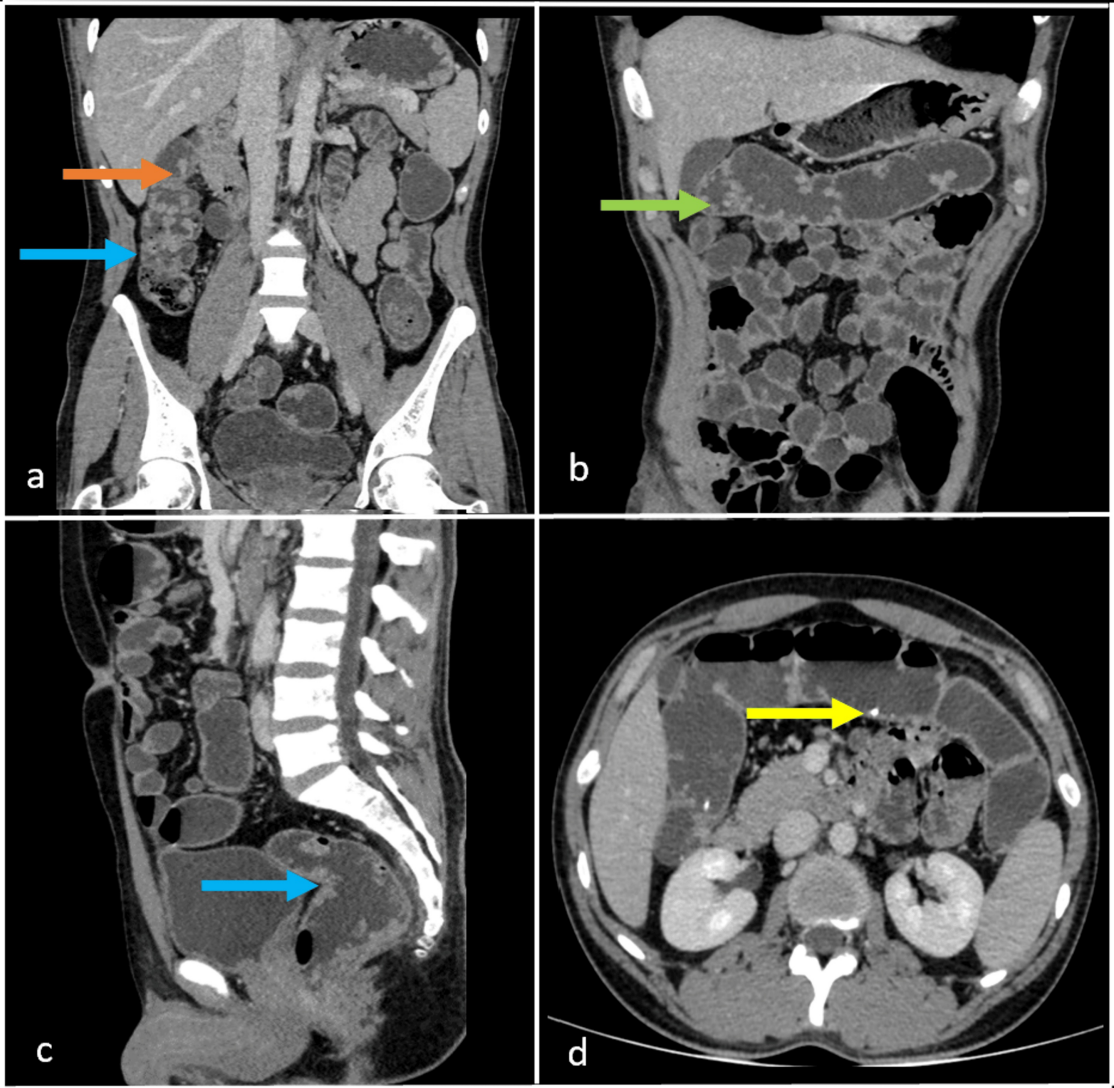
Contrast-enhanced CT abdomen coronal (a and b), sagittal (c), and axial (d) sections of the venous phase show multiple soft tissue polypoidal lesions (blue arrow), both sessile (green arrow) and pedunculated (orange arrow), and a few of them show calcifications (yellow arrow) noted involving the entire large bowel, which on post-contrast shows homogenous enhancement.
